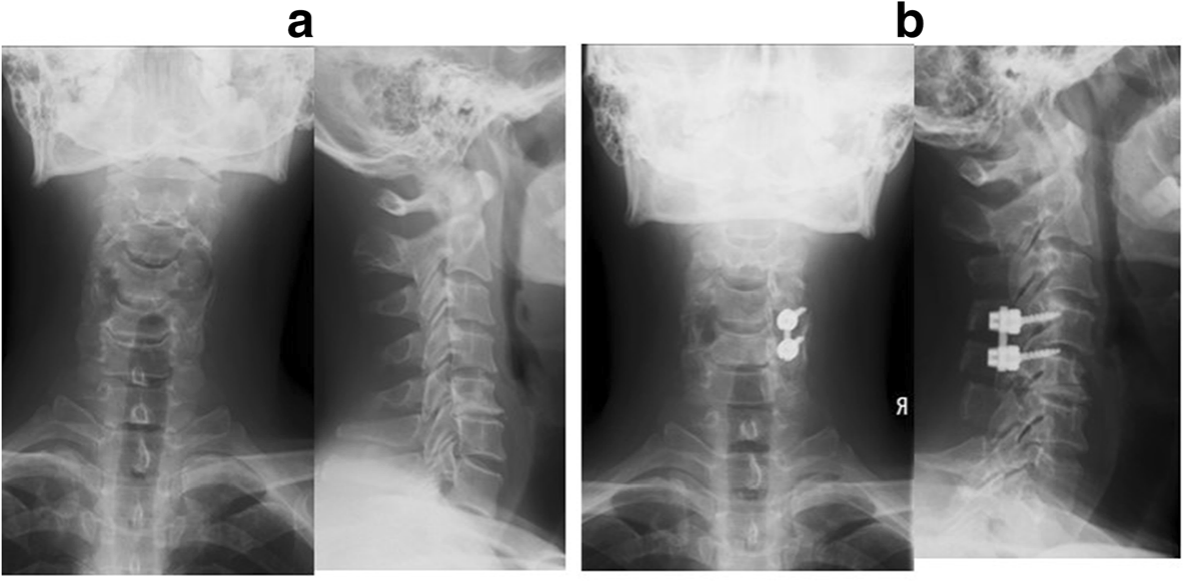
Unilateral lateral mass screw fixation (patient number 11). (a) Plain radiography of the cervical spine of a 54-year-old man showed a positive pedicle sign at the left C5. (b) Plain radiography showed C4 spondylolisthesis (2-mm increase in vertebral slip) which was evident at the last follow-up.
